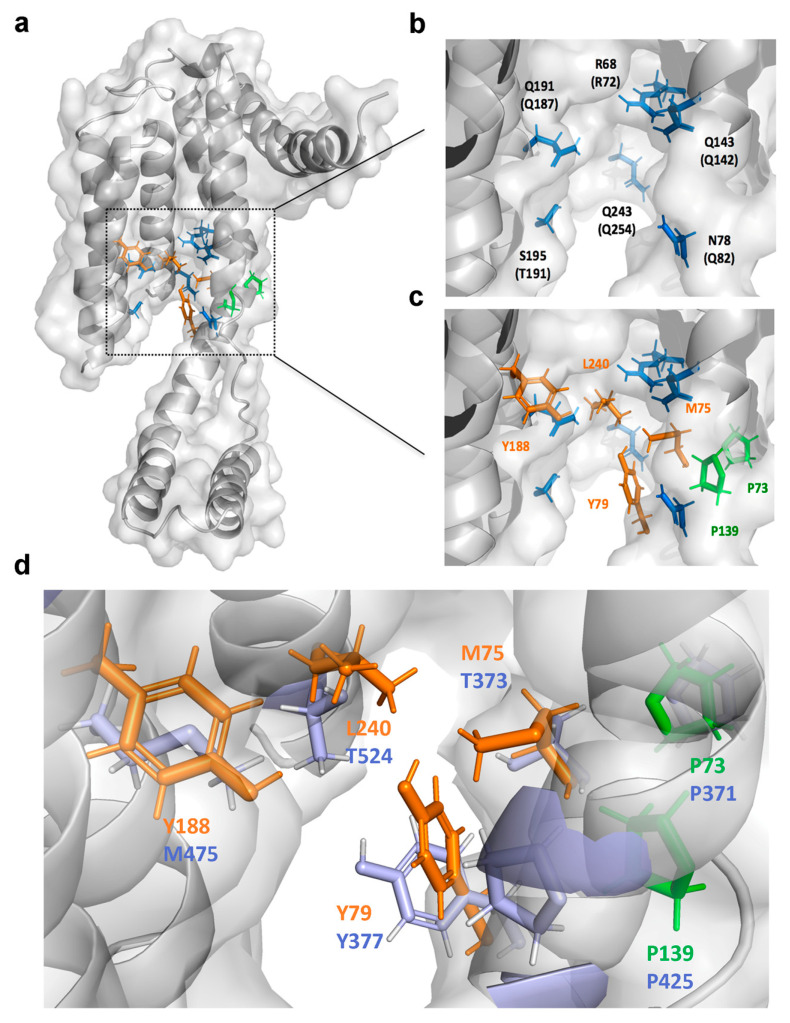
Substrate-contacting cavity of *S. aureus* YidC2. (a) The homology model of *S. aureus* YidC2 was built based on X-ray structures of *B. halodurans* YidC2 (PDB code: 3WO6 and 3WO7). (b) The hydrophilic groove of YidC is found to be conserved and the corresponding resides in *B. halodurans* YidCs are indicated in parentheses. (c) Mutations of YidC2 identified from drug-resistant *S. aureus* isolates are shown in green. The hydrophobic residues in the groove are shown in orange. (d) Comparison of Cpd36-contacting residues of YidC2 in *S. aureus* and *E. coli* shown in orange and purple, respectively.
